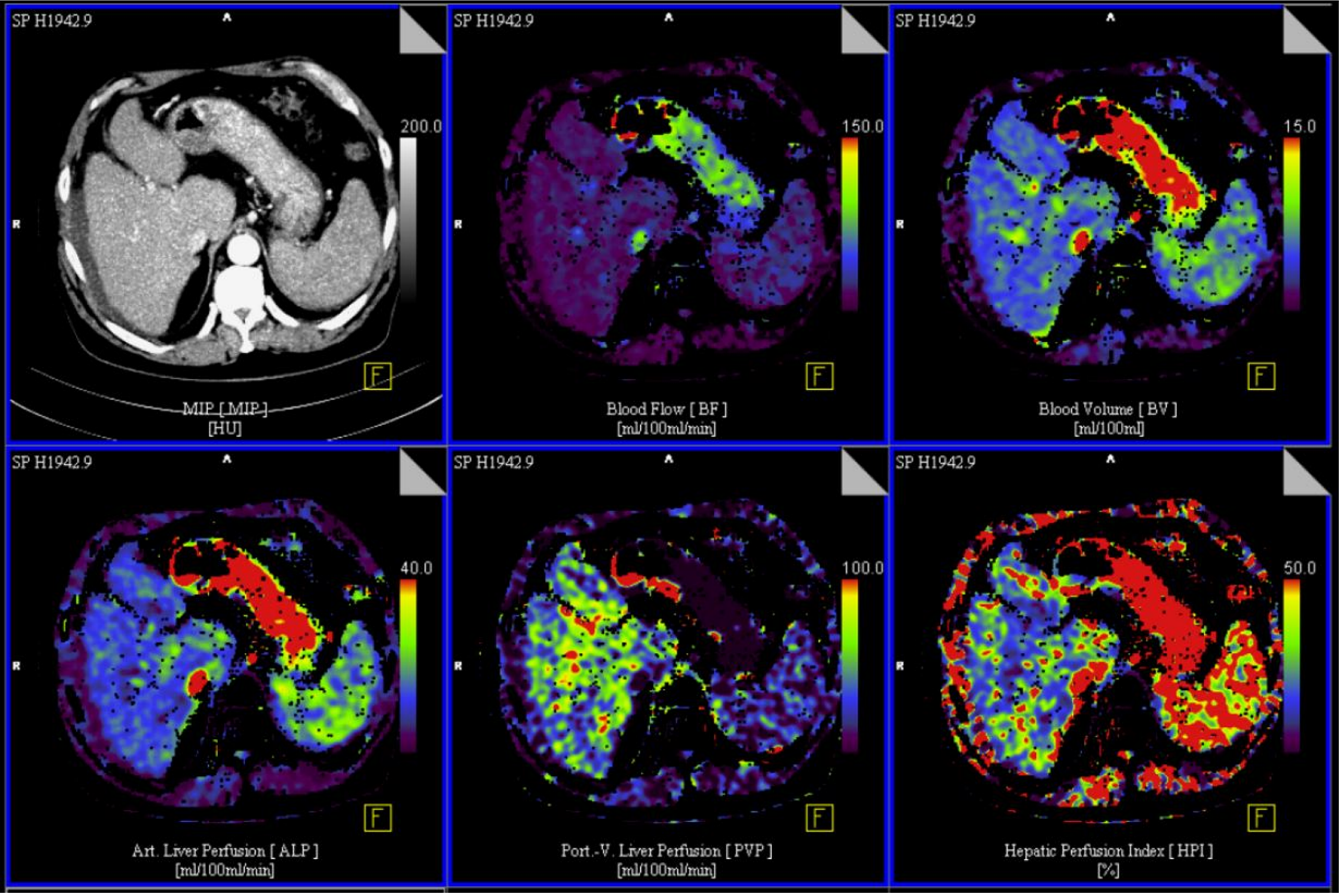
Exemplary axial slides of a perfusion computed tomography of non-tumor liver parenchyma and calculated color maps. Top left: maximum intensity protection. Top center: Blood Flow (BF). Top right: Blood Volume (BV). Bottom left: Arterial liver perfusion (ALP). Bottom center: Portal venous liver perfusion (PVP). Bottom right: Hepatic arterial perfusion index (HPI). In general, red and yellow colors indicate high values, green colors medium values and blue and violet colors low values
